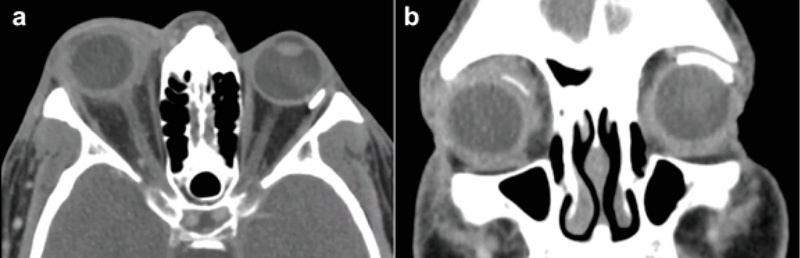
Contrast-enhanced computed tomography of the orbits showing findings consistent with orbital cellulitis, including proptosis of the right globe, thickening of the sclera and optic nerve insertion, small superior fluid collection, and mild anterior retrobulbar fat stranding (A). An Ahmed tube shunt and Baerveldt tube shunt are visualized in the right and left globes, respectively (B).
